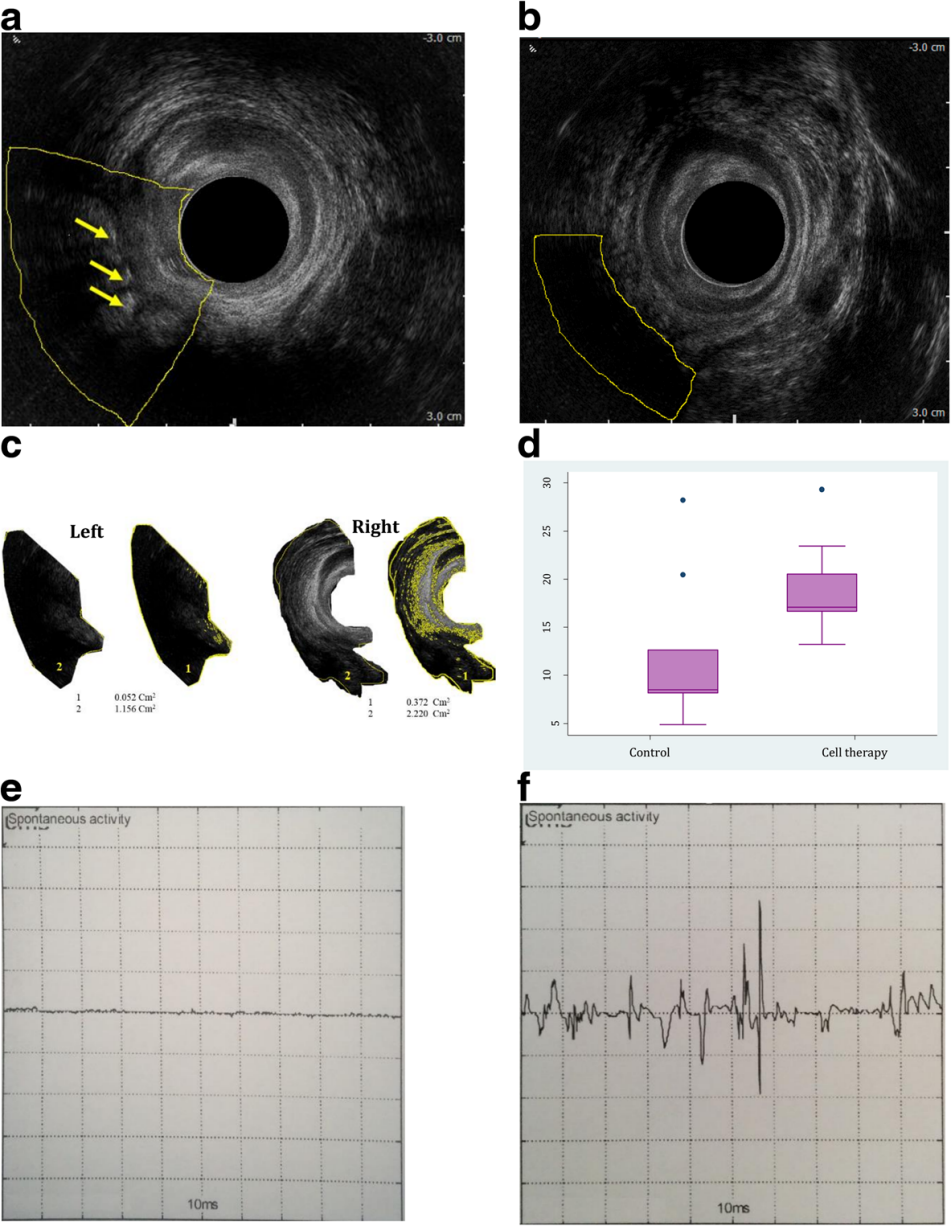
Results of endorectal sonography and EMG 2 months after surgery. a Sphincteroplasty alone, fibrous tissue at the gap of the repair site (*arrows*). b hADSCs + sphincteroplasty, detecting muscle in the gap created in the repair site. c Calculating the ratio of the area occupied by the muscle to total area of the lesion using ImageJ/Fiji 1.46 software (*left*: control group, *right*: cell group). d Median percentage of area occupied by the muscle in the control and cell groups (*p* = 0.02). Sample EMG of the control group (e) and the cell group (f)
